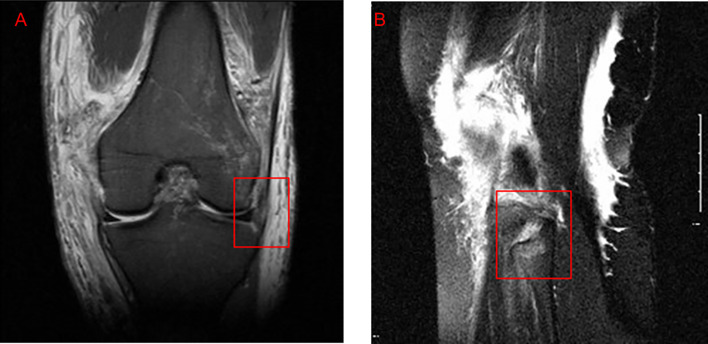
Examples of posterolateral corner (PLC) injuries that were misinterpreted as intact on pre-operative MRI. (A) Coronal fat-suppressed proton-density image shows a full-thickness tear of the iliotibial band (box), reported as intact in the original radiology report. (B) Sagittal proton-density image from a different case that demonstrates disinsertion of the biceps femoris tendon with bone marrow edema of the fibular head (box), also misreported as intact.
